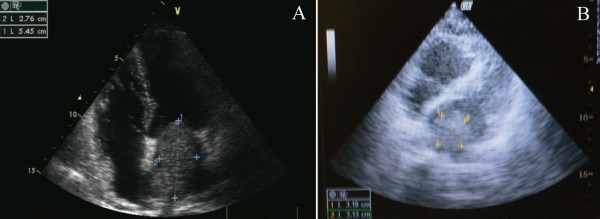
Transthoracic echocardiography revealed a left atrial mass (5.5 × 2.8 cm) before cardiac surgery (A), and a left atrial mass (3.2 × 3.1 cm) five months after surgical resection (B).
